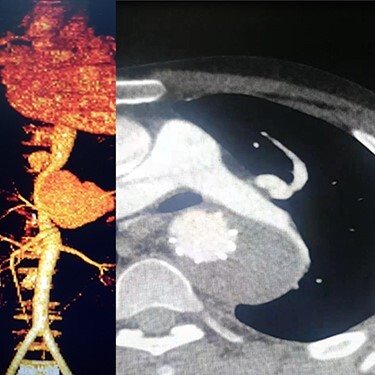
(A) CTA of the left subclavian aneurysm (right), (B) CTA of thoraco abdomen aneurysm (left).
